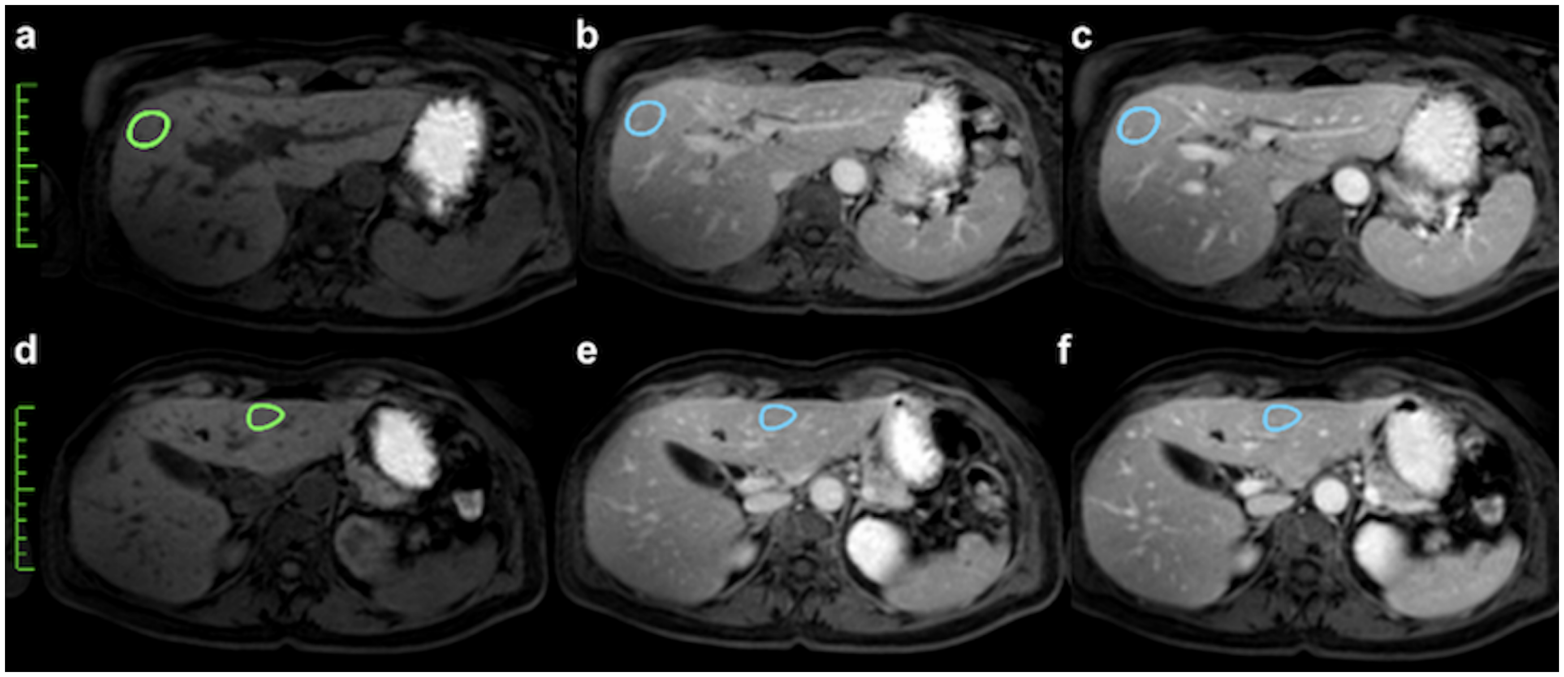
Bolus-timed contrast-enhanced axial T1-weighted breath-hold imaging of a 37-year old female patient. (a) Determination of the relative liver enhancement (RLE) using manually localized regions of interest (ROI) in liver segments of the right lobe (upper row) and left lobe (lower row) according to biopsy location. T1-weighted non-enhanced axial image (a, d); T1-weighted portal venous phase axial image (b, e); T1-weighted delayed phase axial image (c, f).
